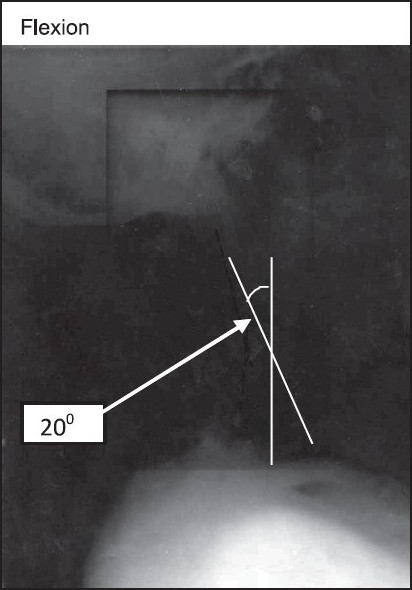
Lateral X-ray of neck with maximum flexion. Maximum flexion achieved was 20 degree
